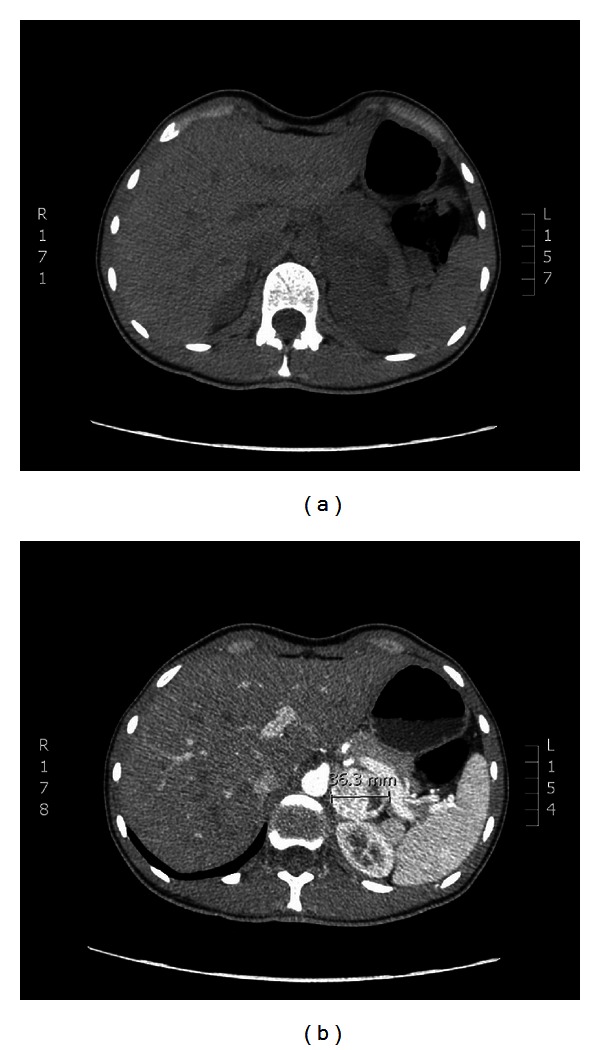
CT scan of the abdomen showing a mass in the left adrenal gland in the noncontrast and contrast series.
